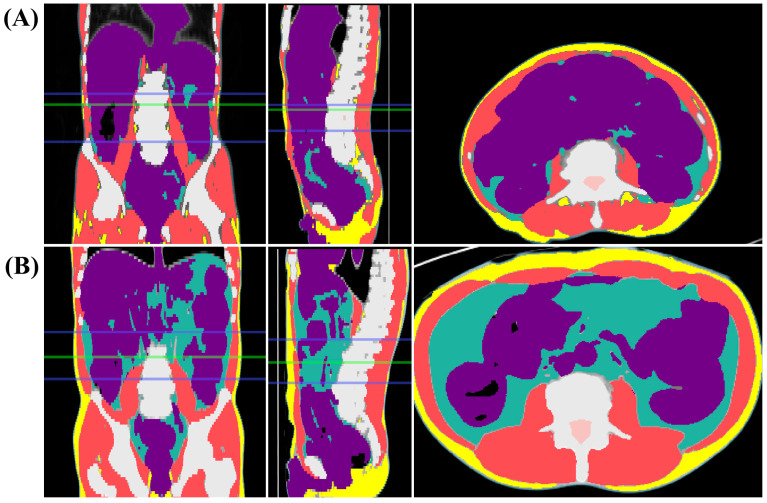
Assessment of body composition through computed tomography (CT) images. (A) CT images of patients classified with volumetric sarcopenia. (B) CT images of patients classified with volumetric non-sarcopenia. The green line marks the L3 vertebral level, while the region between the two blue lines represents the abdominal waist. CT images display skeletal muscle (red), abdominal visceral fat (green), subcutaneous fat (yellow), and visceral organs (purple) within the defined area.
